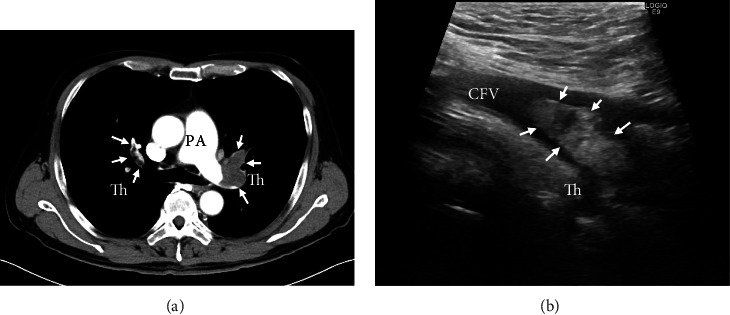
Previous CT and venous echography findings. Previous CT showed bilateral PE (white arrows (a)) and venous echography showed a mobile-shaped thrombus (white arrows) at the proximal site of the left common femoral vein (b). CT: computed tomography, PE: pulmonary embolism, PA: pulmonary artery, CFV: common femoral vein, Th: thrombus.
